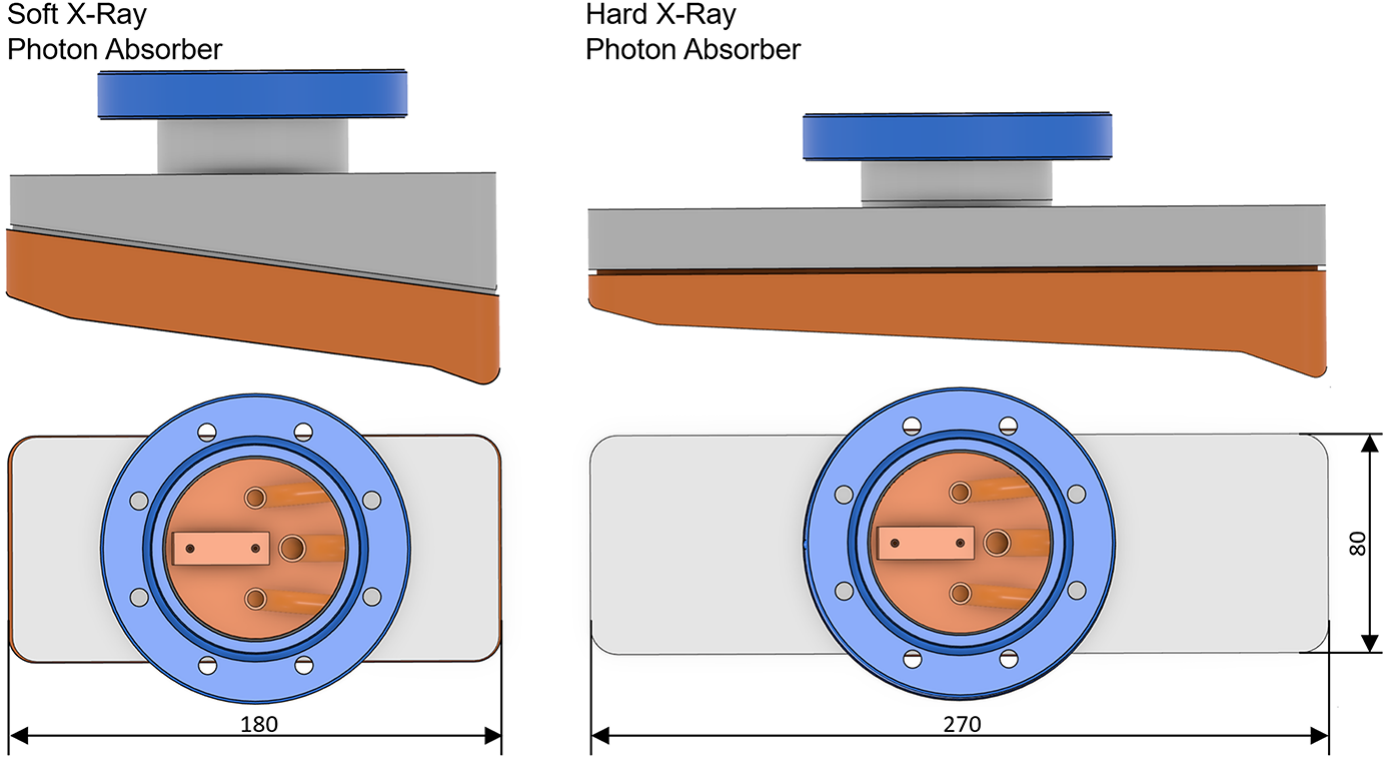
3D model of the two photon absorbers used in the photon shutters. Left: soft X-ray photon absorber. Right: hard X-ray photon absorber.
